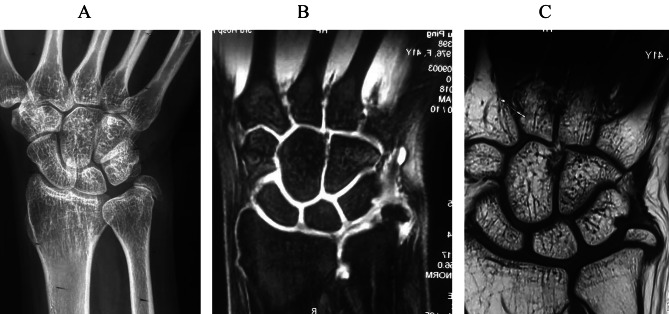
A type 2 ulnar styloid nonunion. (A) X-ray. (B) T1-weighted MRI shows a chondral defect over the triquetrum and intake of the triangular fibrocartilage complex. (C) T2-weighted MRI.
